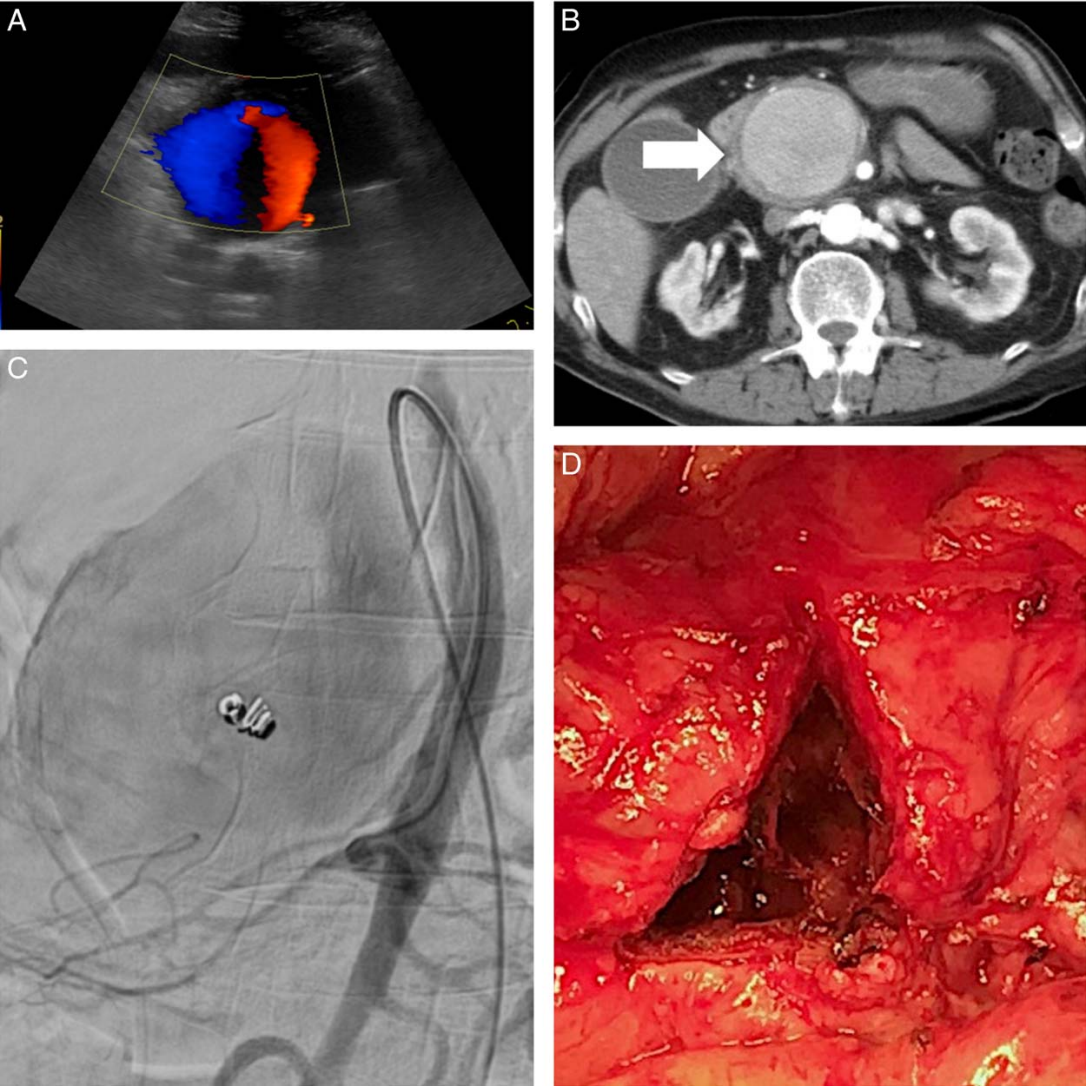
Peri-pancreatic false aneurysm (A) Abdominal sonography showed a cystic lesion with a ‘ying-yang’ sign that signifies a peripancreatic aneurysm. (B) Abdominal CT showed a 6 cm false aneurysm (arrow) arising from the SMA. (C) The angiography showed an SMA aneurysm with two floating micro-coils deployed in a failed coil angioembolization. (D) After ligating the proximal feeding vessel of the aneurysm, we incised the anterior wall of the false aneurysm. We sutured the orifice of the distal feeding vessel from the inner lumen of the aneurysm to stop backflow bleeding.
